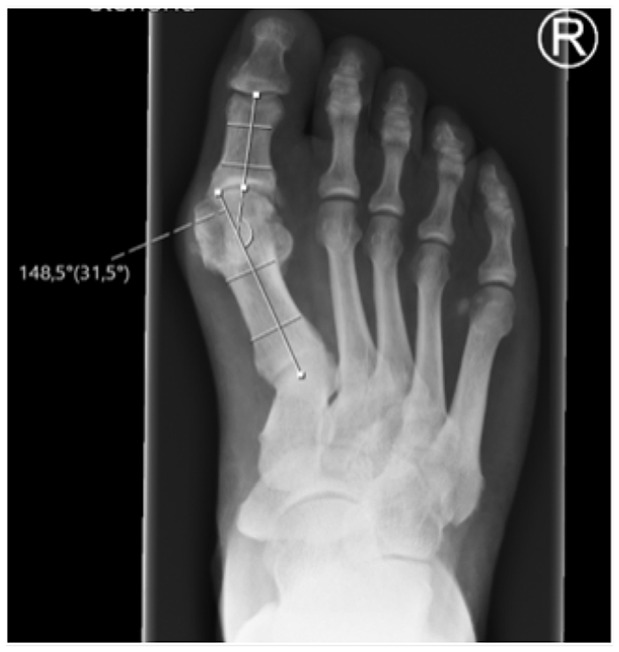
Hallux valgus angle (HVA): Angle between the anatomical axis of the first metatarsal bone and the proximal phalanx on a dorsoplantar radiograph in a standing position with full weight-bearing.
